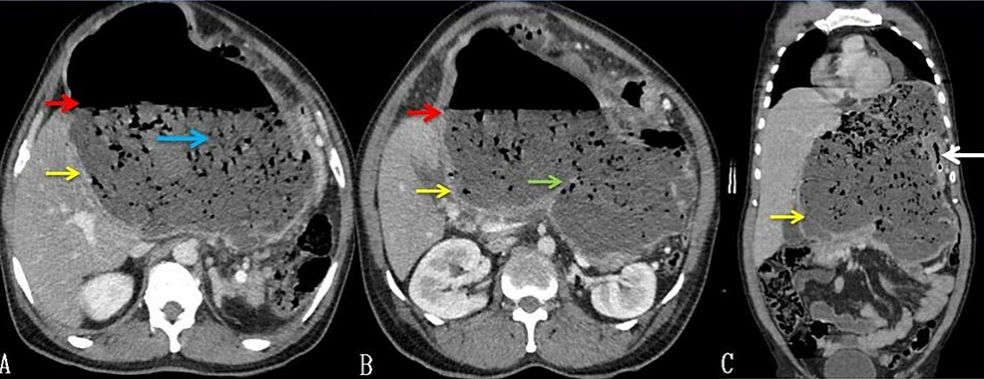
CT of the abdomen (A: axial view, B: axial view, and C: coronal view) A large cyst is seen originating from the left lobe of the liver (yellow arrow) with well defined air-fluid level (red arrow) and debris within the cyst (blue arrow), doubtful septation or membrane (green arrow), stomach being fully compressed (white arrow).
